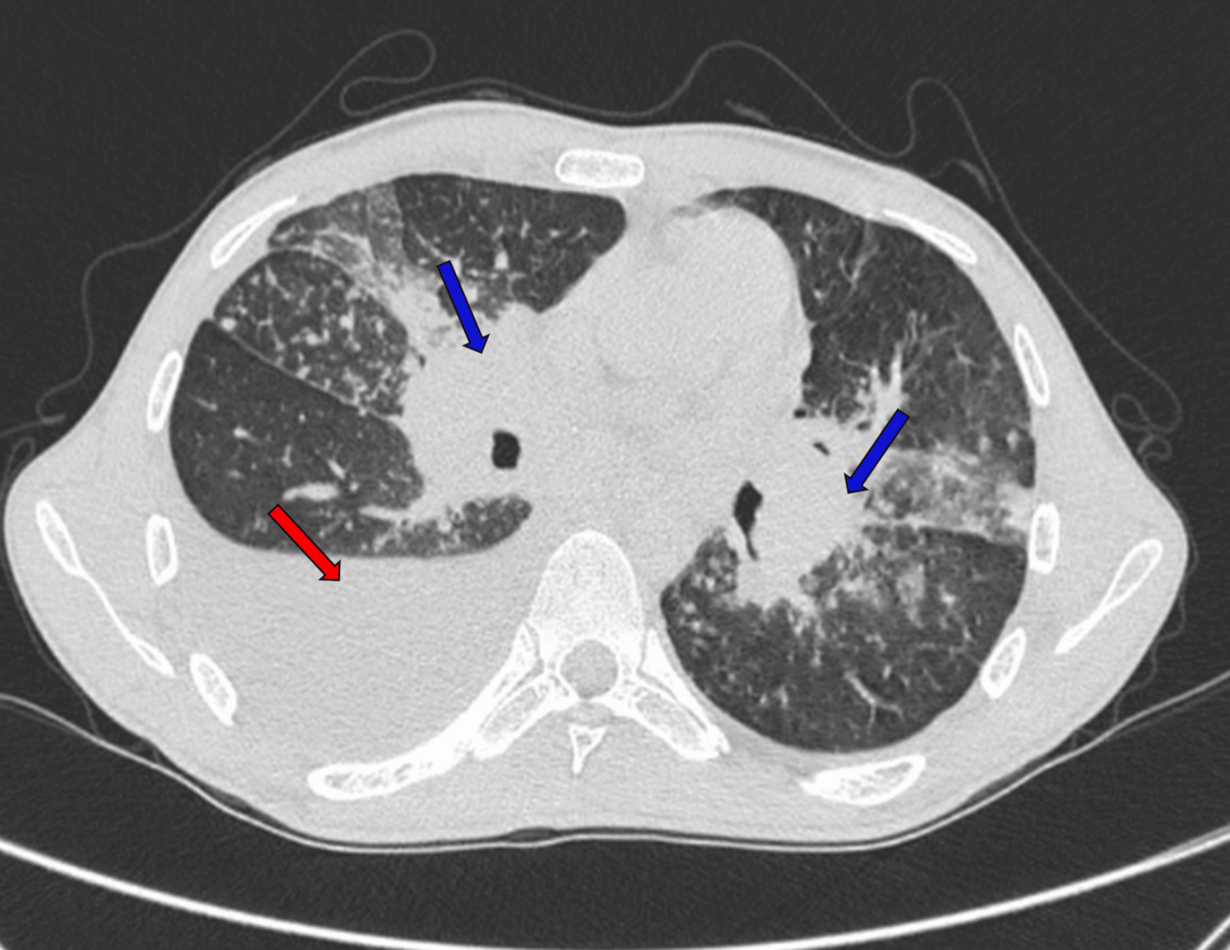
CECT thorax showing right moderate pleural effusion (red arrow) with mediastinal shift to the left side, along with dense consolidations (blue arrows). CECT: contrast-enhanced computed tomography
